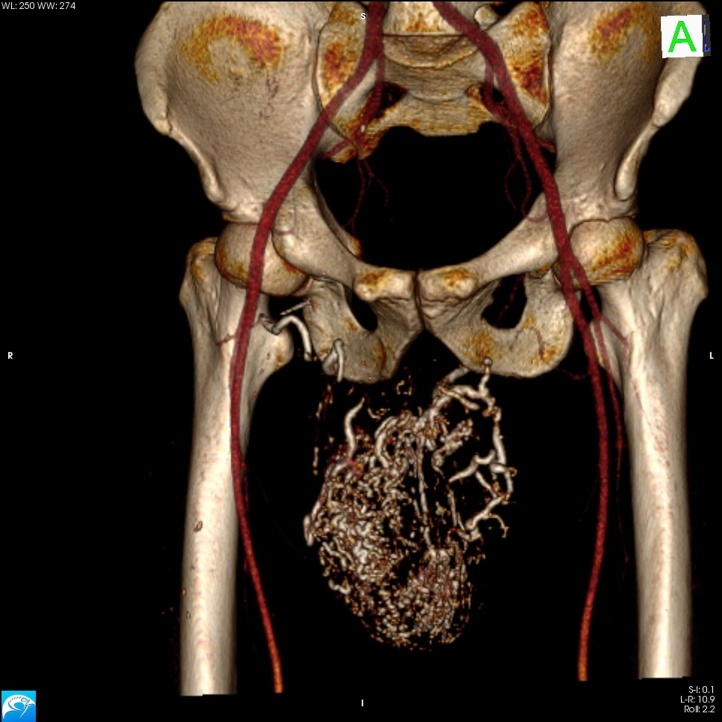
Angio-CT SSD reconstruction swowing normal vasculararisation of the iliofemoral area and the selective embolisation with Glue deposition in the A-V malformation and in the arterial ramifications coming from the right femoral artery.
